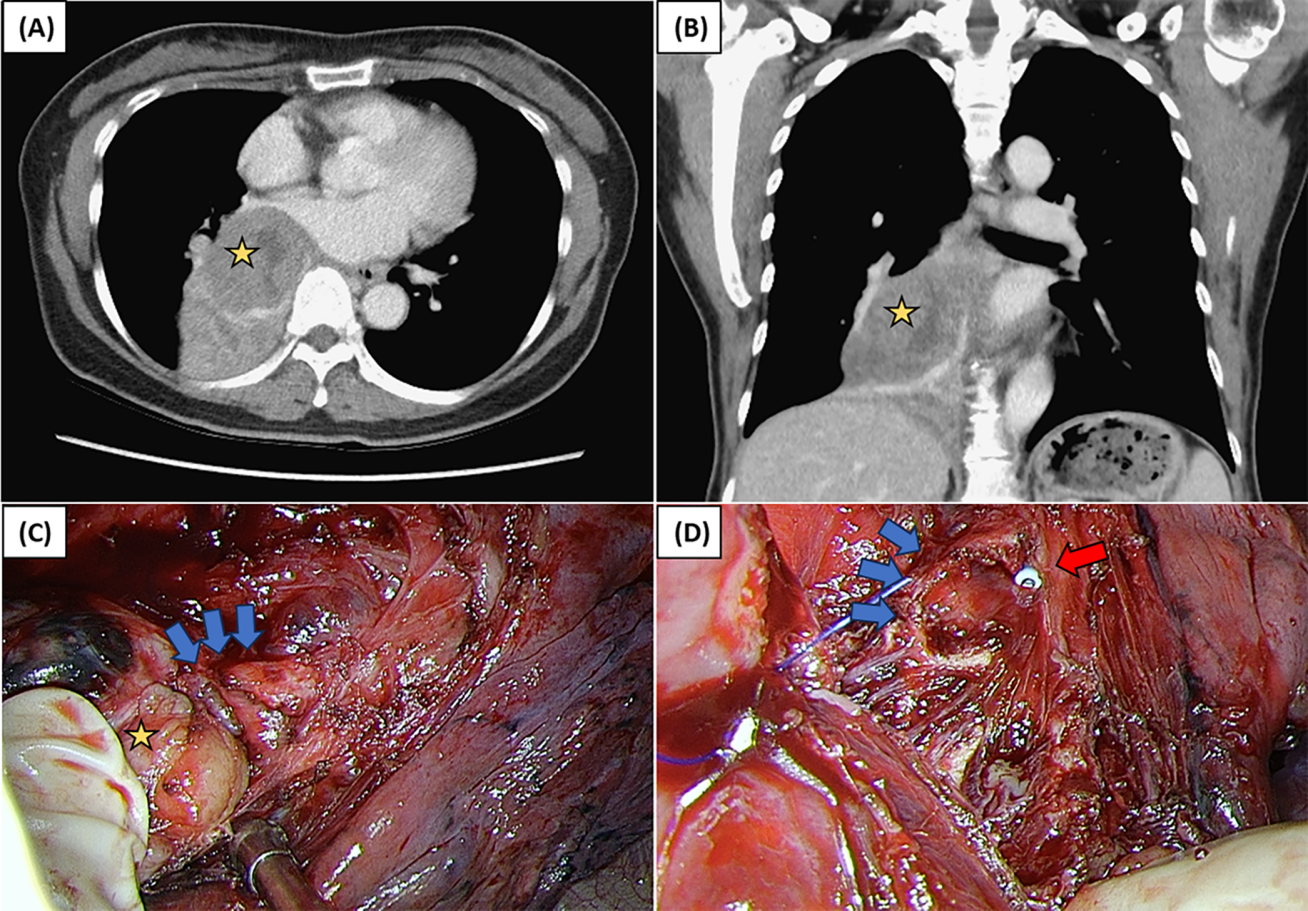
(A) Contrast enhanced computed tomography (CT) axis view: Intra‐bronchial schwannoma (yellow star) with distal obstruction of the right lower lobe. (B) CT coronal view of intra‐bronchial schwannoma (yellow star). (C) Intraoperatively, the tumour (yellow star) was pulled out from the bronchus intermedius (blue arrow). (D) After removal of the tumour, bronchus intermedius (blue arrow) with distal bronchiectasis and the tip of endotracheal blocker (red arrow) were seen.
